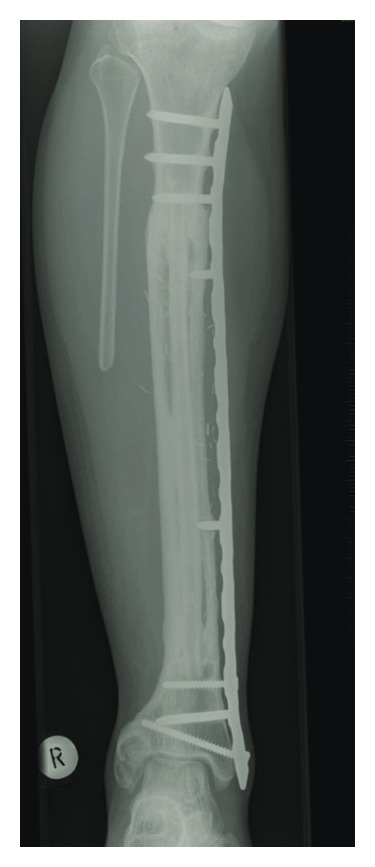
Postoperative AP radiograph at the time of 5-year followup. Surgery was performed due to multicentric adamantinoma of the right tibia and fibula. The tibial defect was bridged with a homologous tibia allograft and a vascularised fibula autograft.
